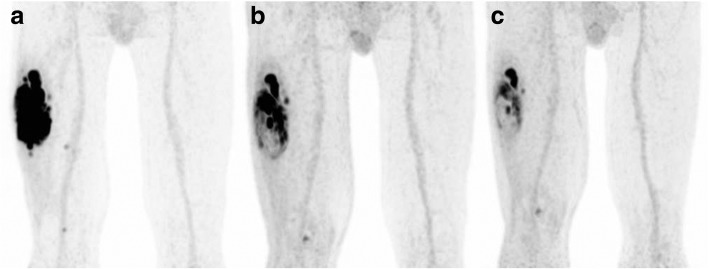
^18^F-FDG uptake throughout the tumor for one of the patients during the treatment course. Coronal ^18^F-FDG PET-CT images showing the heterogeneous ^18^F-FDG uptake throughout the tumor for one of the patients during the treatment course. a Scan 1 (baseline). b Scan 2 (after HILP). c Scan 3 (after EBRT)
